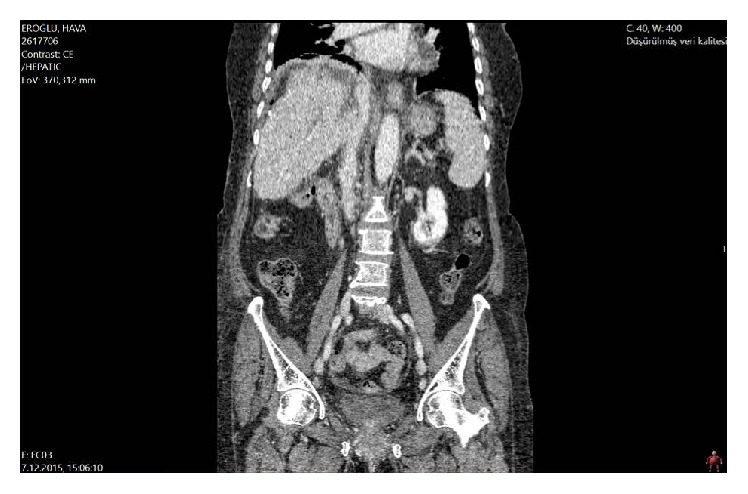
The postoperative sagittal section of the abdominal CT. IVC is seen as patent.
